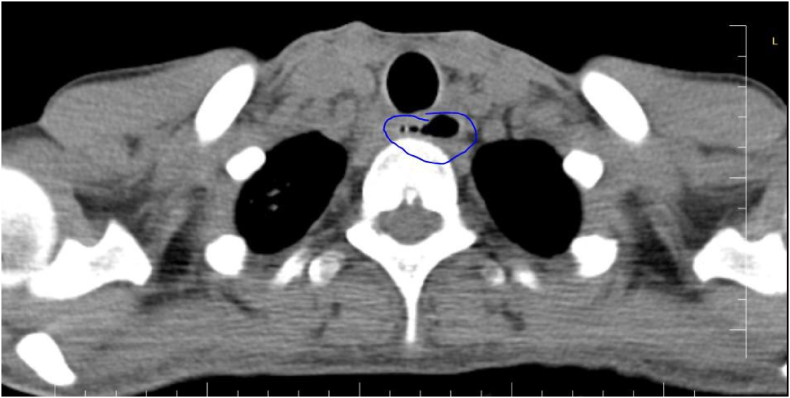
Axial chest CT shows fistulate tract, which contains air and communicates with the esophagus.
